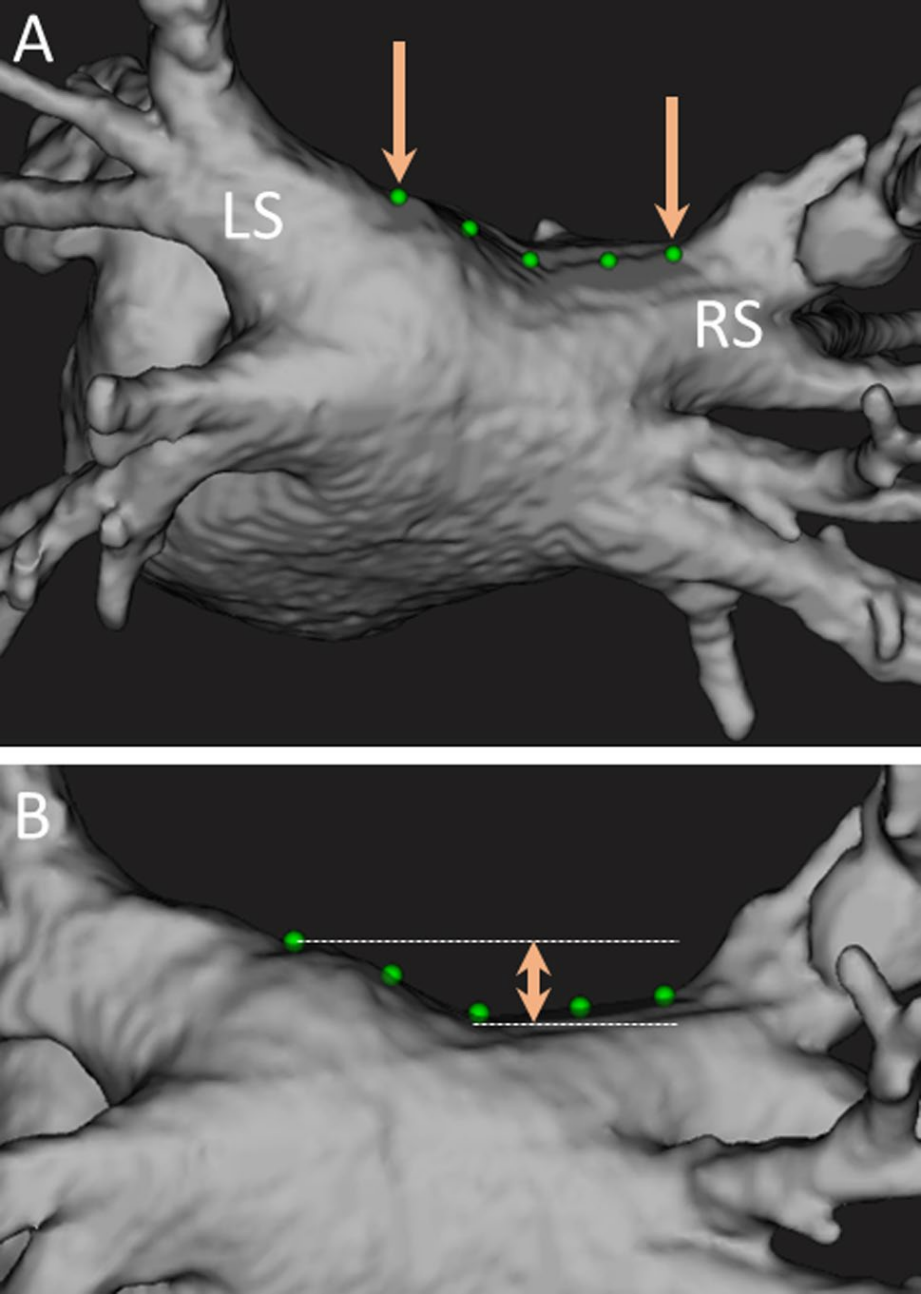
Measurement for roof line length (A) and height (B). A The inflection points of the left and right superior pulmonary veins and left atrium are first identified as indicated by the arrows. Three points on the LA roof are chosen between these inflection points, and the distance drawn between them is measured as the roof line length. B The height of the roof line is determined by measuring the distance between its highest and lowest points on the posterior anterior view CT imaging. LS, left superior pulmonary vein; RS, right superior pulmonary vein; LA, left atrial; CT, computed tomography
